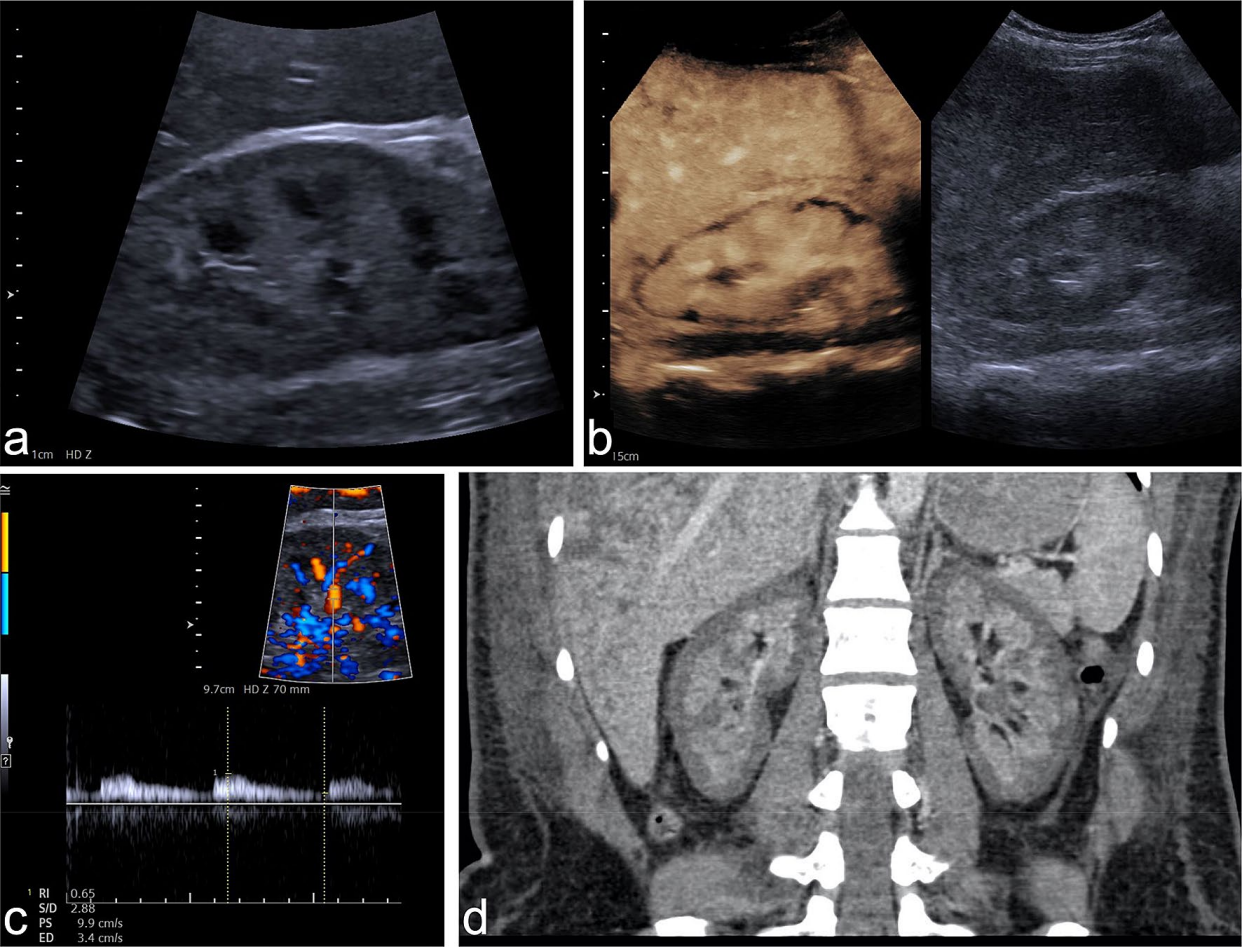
Example of RCN of the native kidney in a 28-year-old woman suffering from massive postpartum bleeding with acute kidney failure and HELLP syndrome. (a) B-mode image of the right kidney showing a hypoechoic rim of 3–4 mm. (b) CEUS of the right kidney showing a subcapsular loss of contrast enhancement of 3–5 mm. (c) Triplex sonography of the right kidney with a PW spectrum of an interlobar artery showing a normal resistance index of 0.65. (d) Coronal venous-phase CT scan obtained 14 days before CEUS examination showing a recess of contrast agent measuring up to 6 mm in both kidneys, confirming the diagnosis of RCN. CEUS denotes contrast-enhanced ultrasound, RCN, renal cortical necrosis, HELLP syndrome denotes hemolysis, elevated liver enzymes, low platelets, PW, pulsed-wave Doppler.
